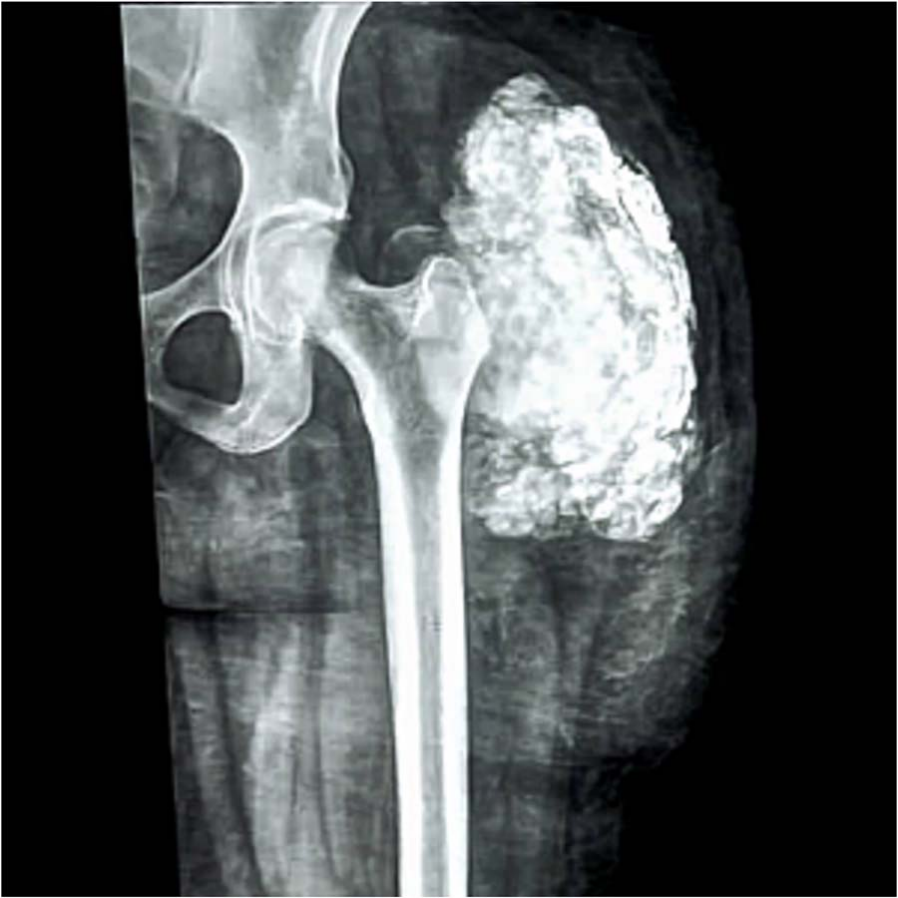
The X-ray of the left upper thigh and hip shows a calcified mass with a well-defined margin (“chicken wire” pattern calcification).
